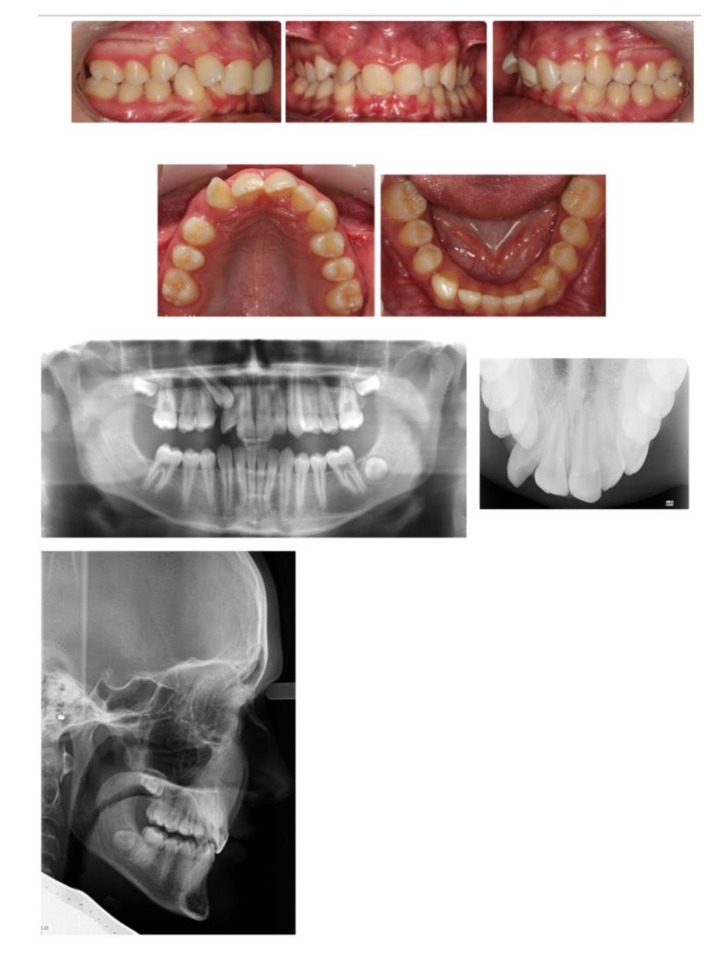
Pre-treatment intraoral photographs and radiographs. The extraction of all molar incisor hypomineralisation (MIH)-affected FPMs was carried out at the age of 9. Note the congenitally absent LR8.
